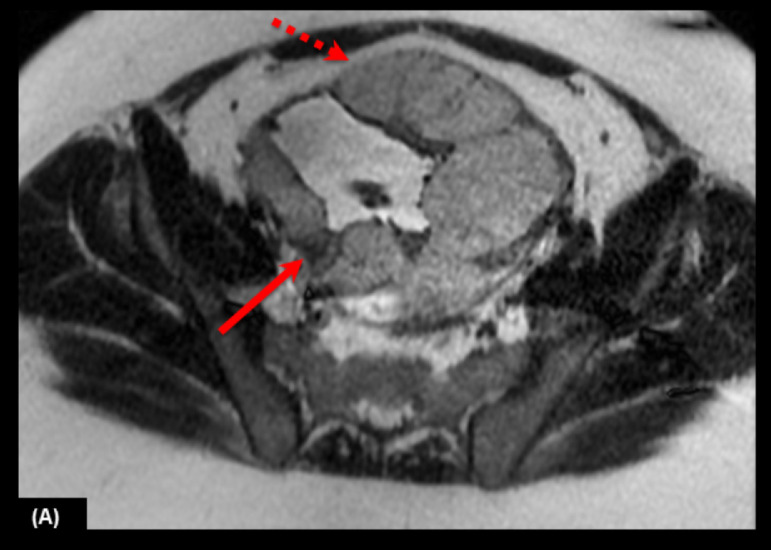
42-year-old woman at 35 weeks' gestation and one previous C-section. (A) Axial T2 weighted images shows intraplacental T2 dark band (arrow) and placental bulges (dashed arrows). - 5: > 20 mm - 4: 15-20 mm - 3: 10-14 mm - 2: < 10 mm - 1: absent2- Placental bulge: focal bulging of the uterine contour.[14,19,24,27] - 5: clear loss of myometrial congruency or invasion of adjacent organs - 4: focal bulges - 3: disruption of the normal pear shape of the uterus with the lower uterine segment being wider than the fundus - 2: slight irregularities on uterine contours - 1: normal contours3- Placental protrusion sign: placenta pushing inferior to the internal os.[21] - 5: > 15 mm - 4: 11-15 mm - 3: 6-10 mm - 2: < 5 mm - 1: absent4- Myometrial thinning: focal thinning and indistinctness of the myometrium and loss of thin dark uteroplacental interface on T2-weighted images.[18,28,29]5- Heterogeneous placenta: marked heterogeneous intensity within the placenta (Fig. 5).[18,30, 31, 32]
